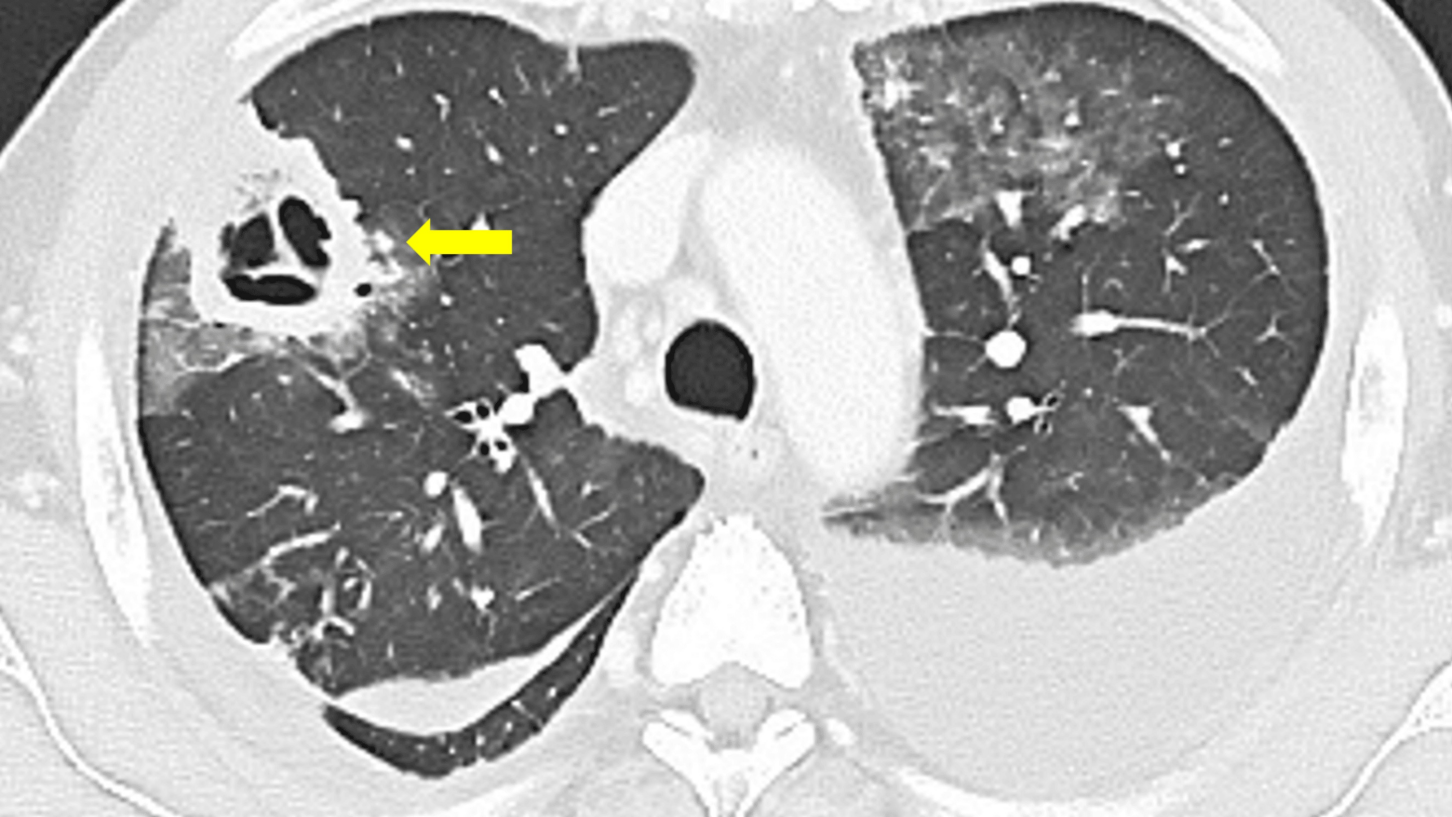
Chest computed tomography (CT) on hospital day 3 demonstrating cavitary change in the mass and bilateral pleural effusion (yellow arrow).
